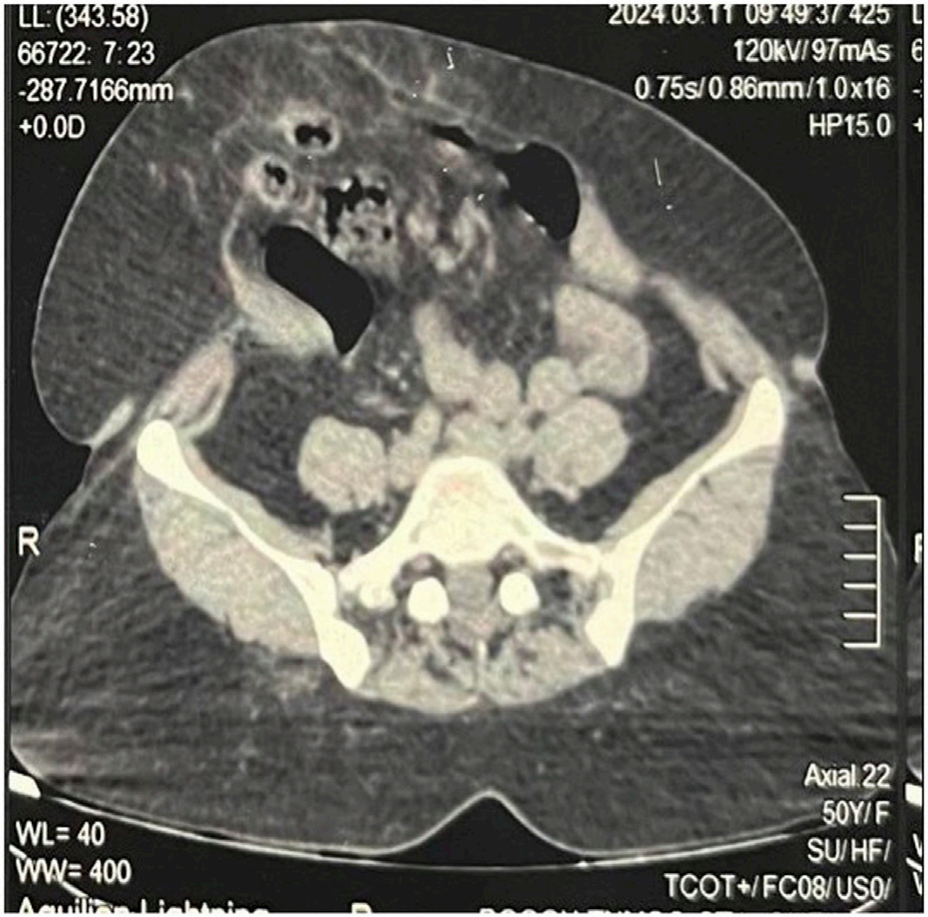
Pre BT CT Abdomen showing tense and shortened abdominal wall muscles.
